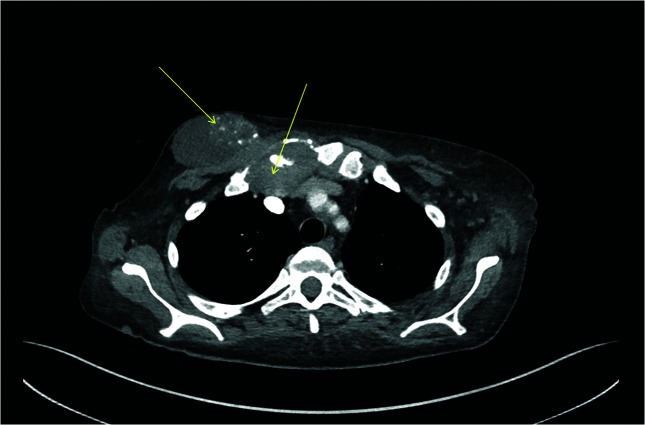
Computed tomography (CT) thorax showing the sternoclavicular mass as indicated by the two arrows.
